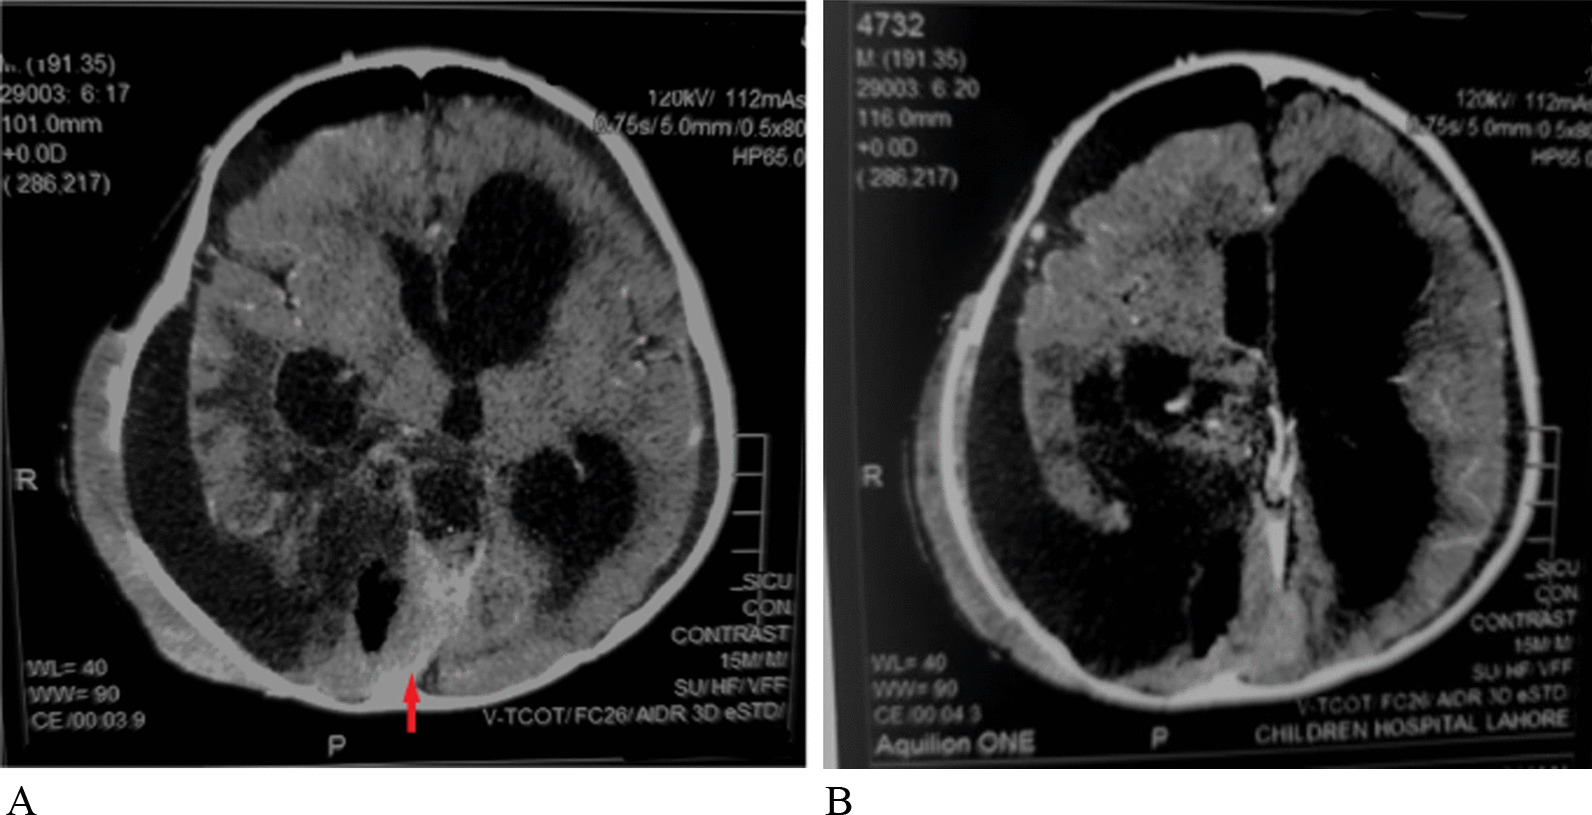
A, B Postoperative CT without contrast: A major bulk of the tumor had been removed with a small enhancing dural residual mass (2.9 × 1.3 cm on axial images) adherent to posterior interhemispheric fissure (red arrows).
